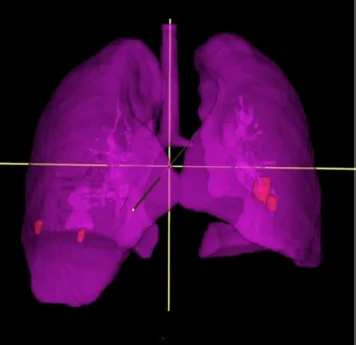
Three-dimensional chest CT reconstruction demonstrating multiple bilateral pulmonary metastases from undifferentiated uterine sarcoma.
